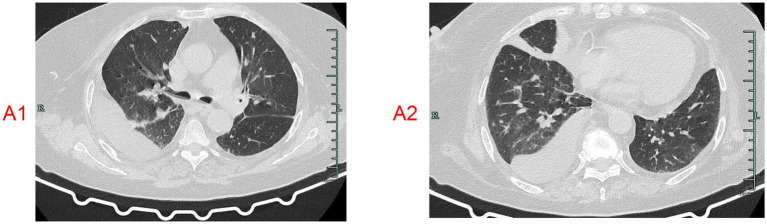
(A1–E2) Changes in chest CT during the entire treatment process.
